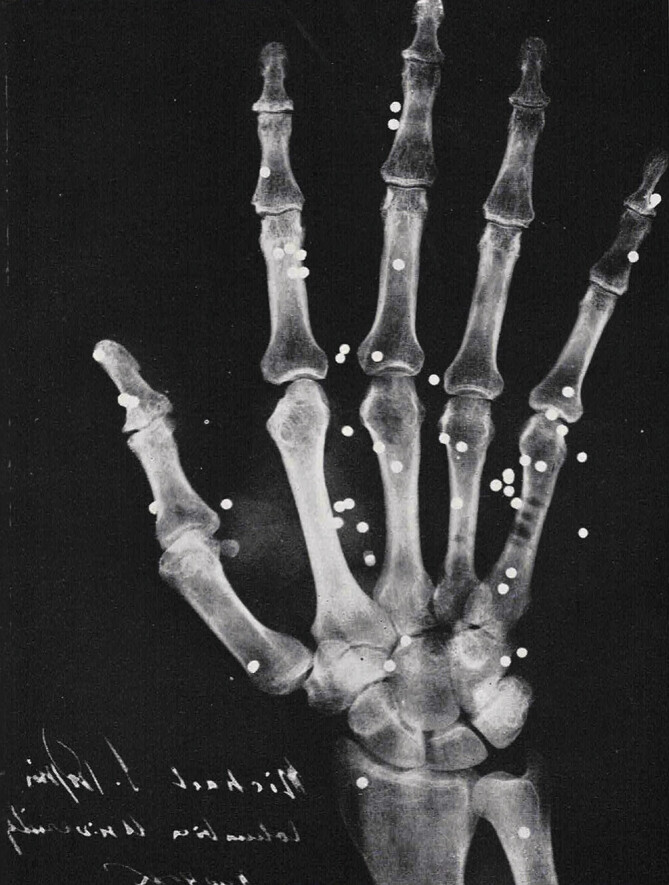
X-ray of the left hand of an anonymous individual, signed by Michael I. Pupin. Reproduced as published. Brecher R. and E. The Rays. A History of radiology in the United States and Canada. Baltimore,The Williams and Wilkins Company,1969:Plate VI. [rerif]
